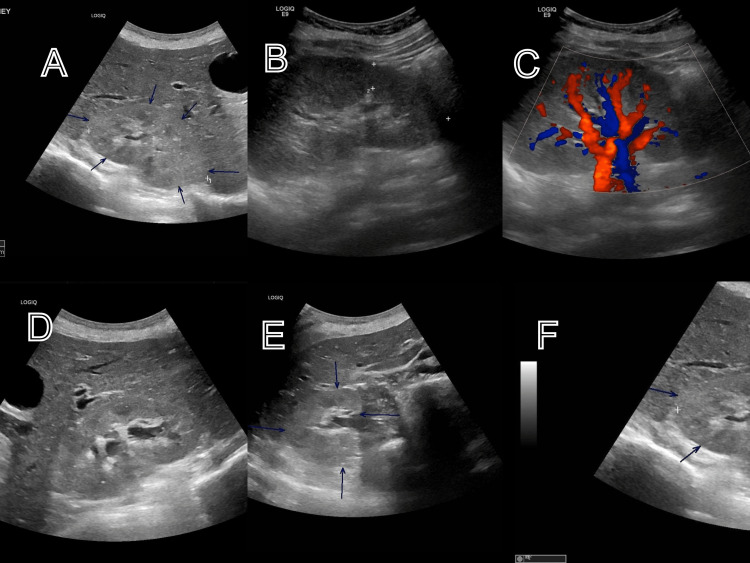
Renal ultrasound with arrows showing some features of pyelonephritis A: loss of corticomedullary differentiation, mixed echogenicity with mild enlargement; B: renal enlargement; C: increased renal perfusion on Doppler; D: modest caliceal dilatation (hydronephrosis); E: transverse plane showing accentuated cortical echogenicity; F: comparison of the echogenicity of the liver and kidneys and kidney outline loss from edema.
